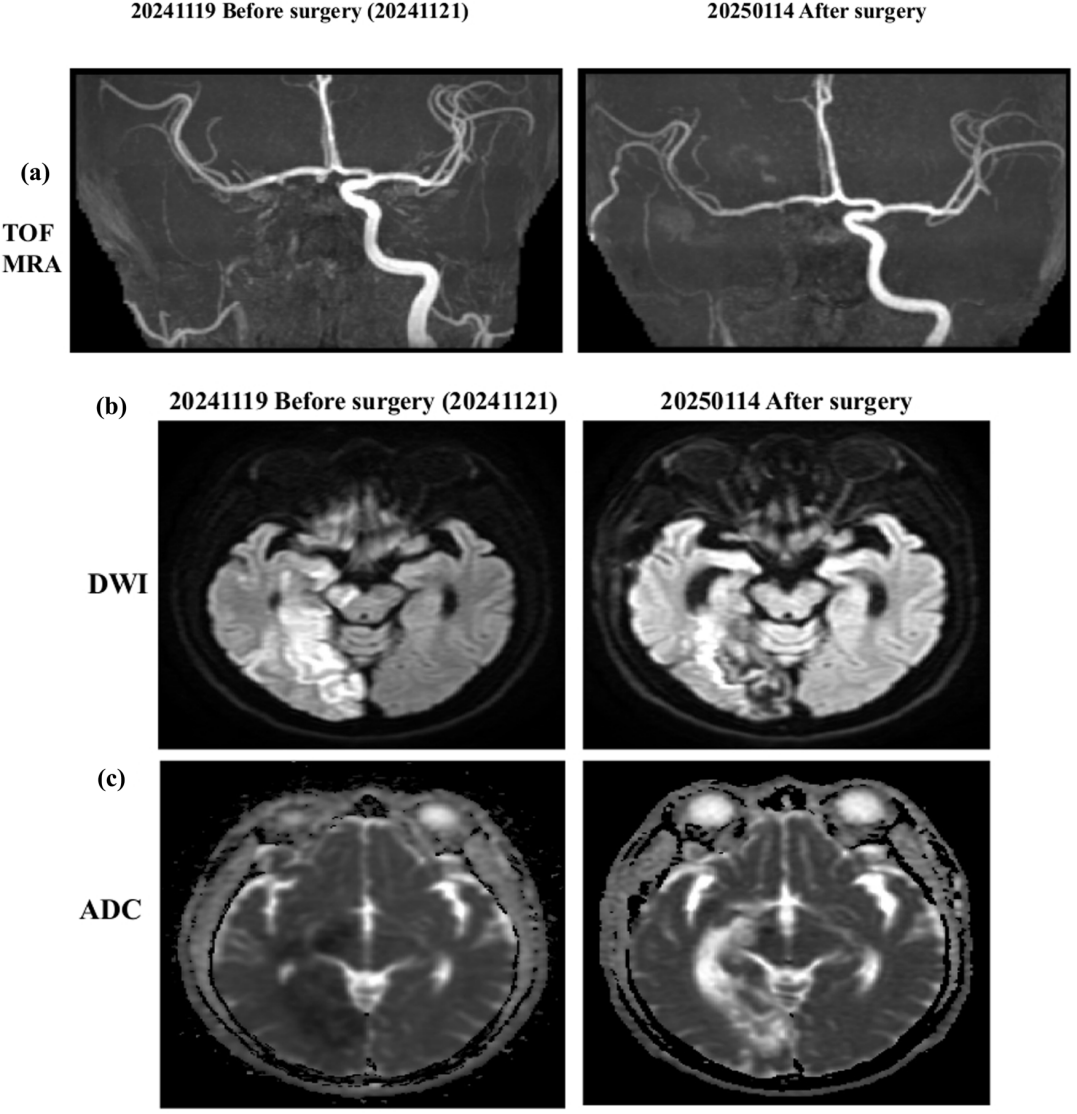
Serial neuroimaging before and after STA–MCA bypass surgery. (a) TOF MRA performed on November 19, 2024 (preoperative), demonstrates occlusion of the right ICA with reduced distal perfusion. Follow-up MRA on January 14, 2025 (postoperative), shows improved collateral circulation in the MCA territory, consistent with successful revascularization via STA–MCA bypass. (b) Diffusion-weighted imaging (DWI) prior to surgery reveals hyperintense signals in the right hemisphere, indicating acute ischemic changes. Postoperative DWI shows no new hyperintensities, suggesting the absence of additional infarcts. (c) Apparent diffusion coefficient (ADC) maps demonstrate corresponding preoperative hypointensities, confirming restricted diffusion in infarcted regions. Postoperative ADC findings reflect infarct maturation without evidence of extension.
